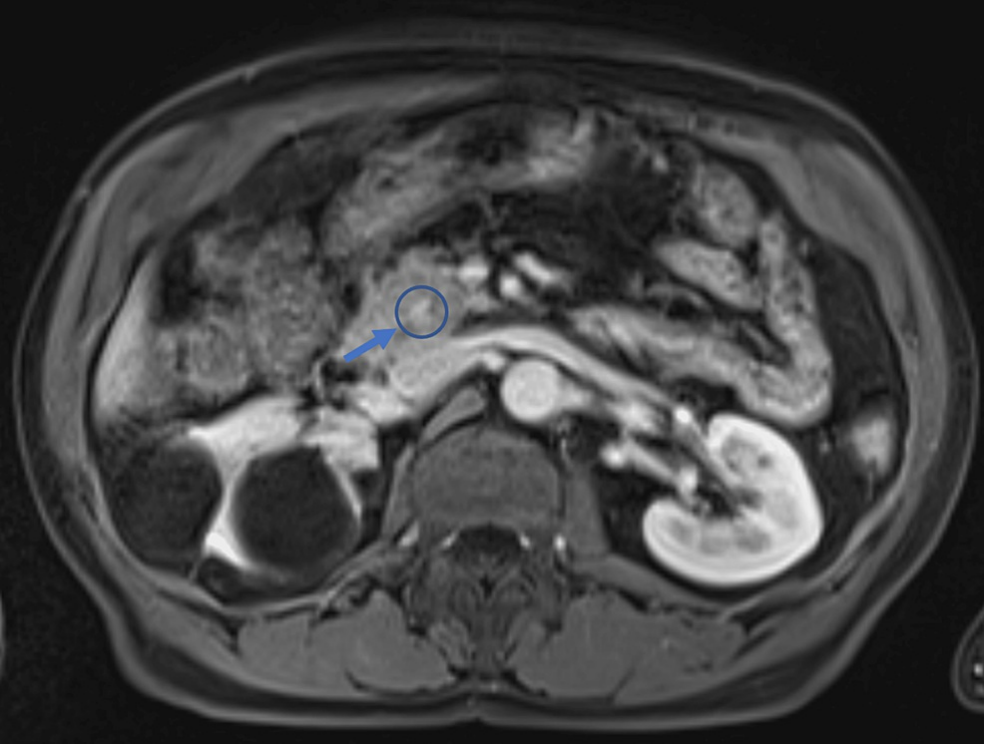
MRI showing a nodular area in the cephalic region of the pancreas with 10 mm that could represent a neuroendocrine tumor. MRI: magnetic resonance imaging.
